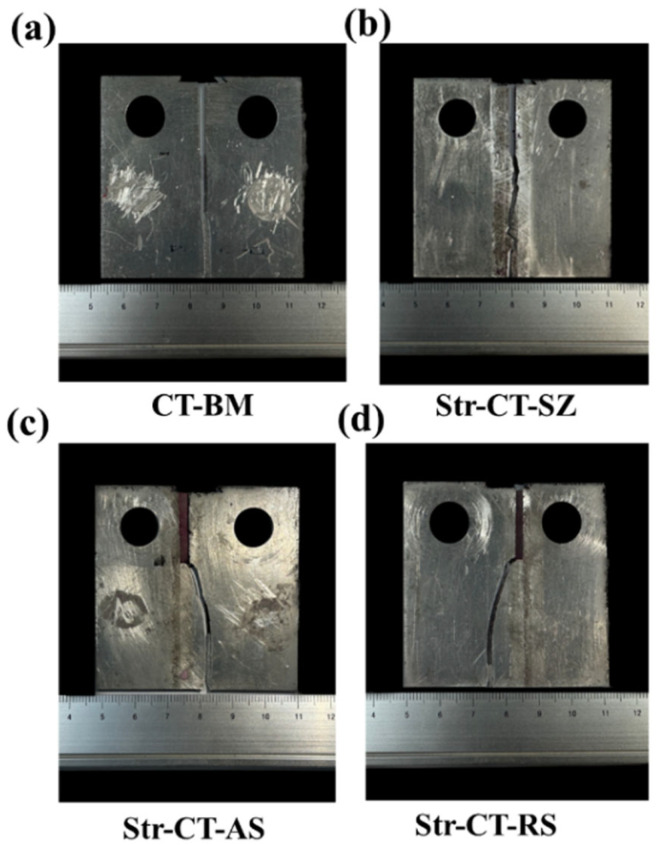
The morphologies of CT samples after FCG test: (a) the morphology of CT-BM, (b) the morphology of Str-CT-SZ, (c) the morphology of Str-CT-AS and (d) the morphology of Str-CT-RS.
